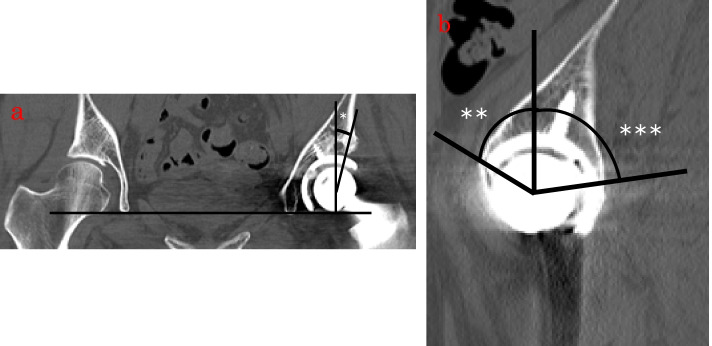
Postoperative computed tomography of THA. The LCE angle (*) is defined as shown in the postoperative coronal computed tomography image of THA (a). The ACE () and PCE angles (*) are defined as shown in the postoperative sagittal computed tomography image (b) THA: total hip arthroplasty; LCE: lateral cup center-edge; ACE: anterior cup center-edge; PCE: posterior cup center-edge
